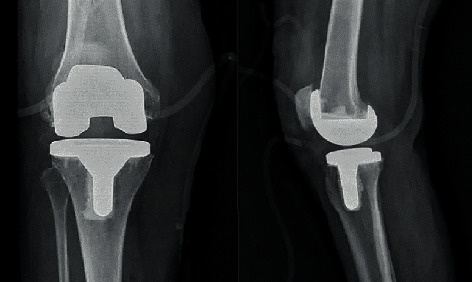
Total knee replacement X-ray [70].
